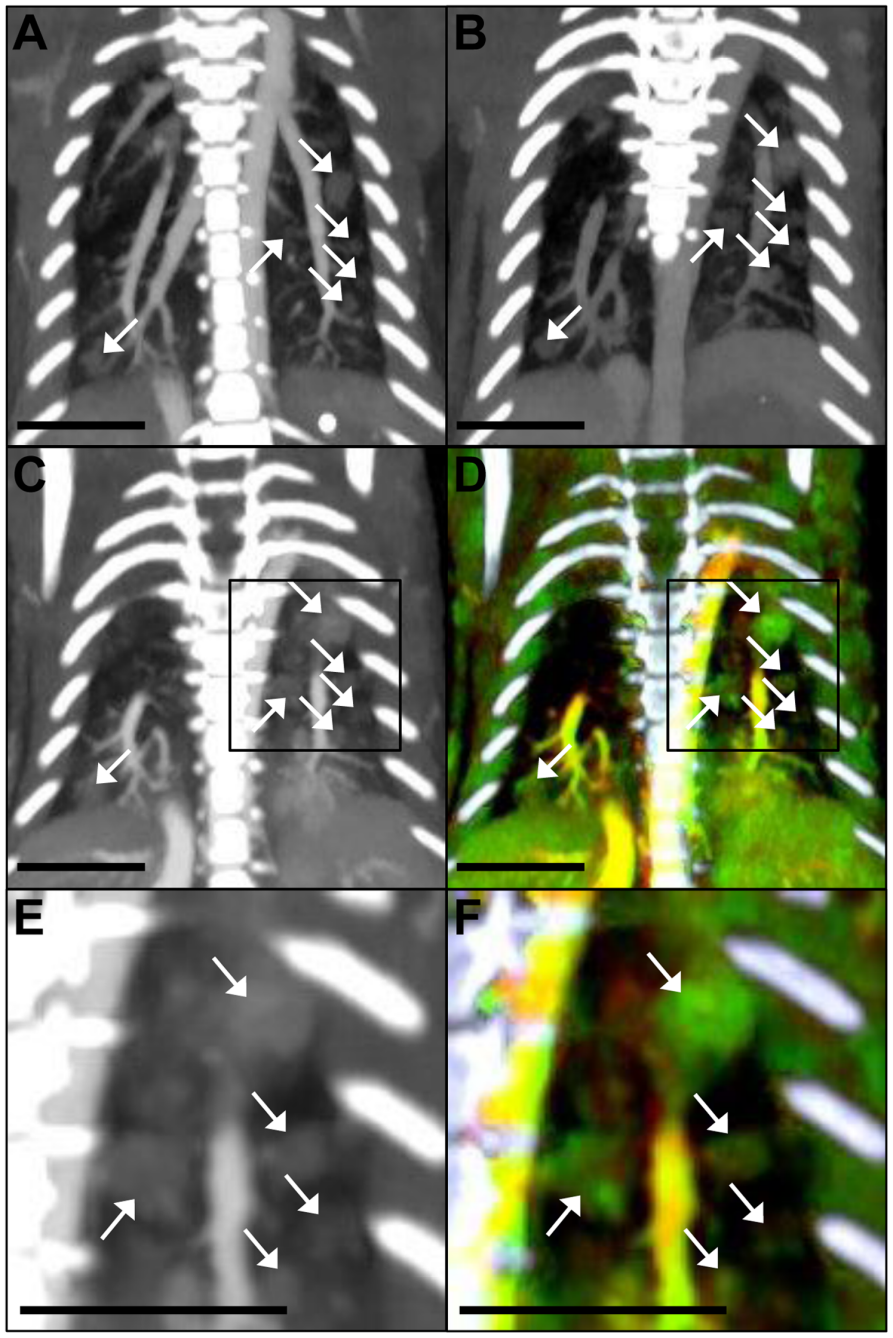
Thick slab maximum intensity projections of the lungs. Coronal CT images showing the filtered 80 kVp dataset from CT scan 1 (A), CT scan 2 (B), CT scan 3 (C), and the final overlay of the DE decomposition of CT scan 3 (D) with bones segmented out (bones in white). (E) is a zoomed-in image of the right side of CT scan 3, and (F) is a zoomed in image of the right side of the DE overlay of CT scan 3. The boxes in (C) and (D) show the regions that are enlarged in (E) and (F), respectively. 80 kVp images are windowed from –300 to 1200 HU. The overlays are windowed from 0.25 to 6 mg/mL gold, and 0.25 to 15 mg/mL iodine. The scale bar represents 0.5 cm in all images.
